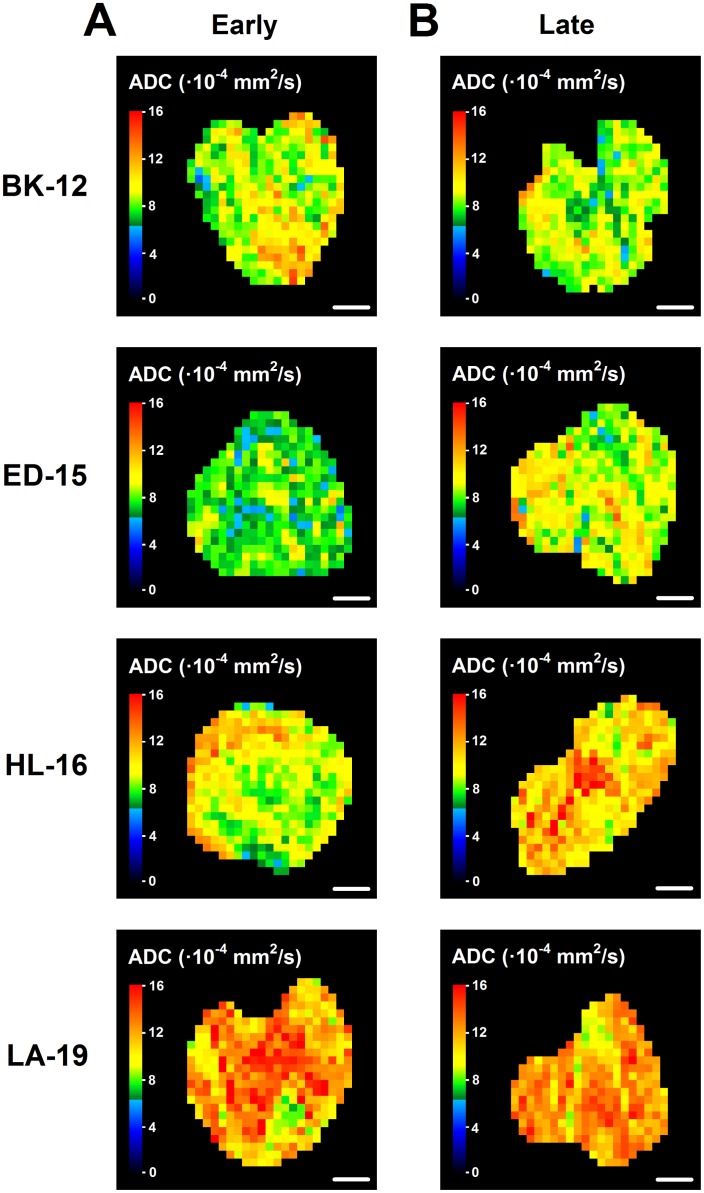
Diffusion-weighted magnetic resonance imaging-derived parametric images ADC (apparent diffusion coefficient) images of early (A) and late (B) generation BK-12, ED-15, HL-16, and LA-19 tumors. Scale bar: 2.0 mm.
